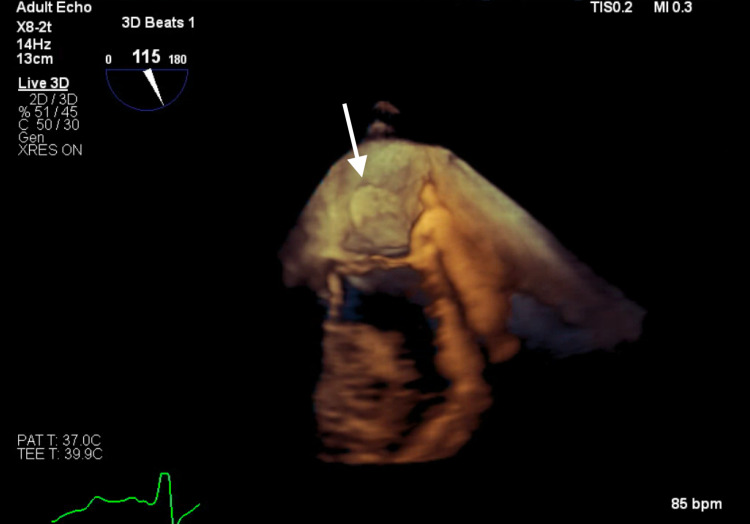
Transesophageal echocardiogram 3D volume data set cropped over the left atrium to reveal the extent of the left atrial wall calcification.
